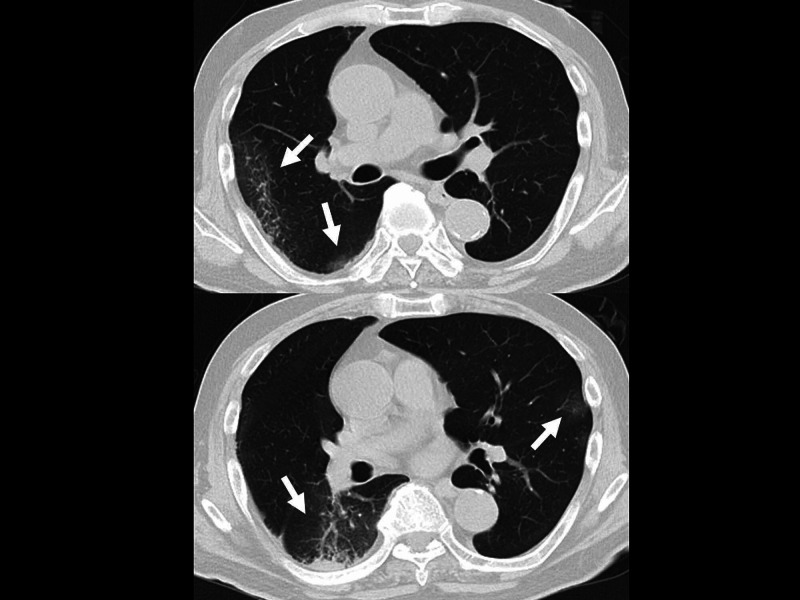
Thoracic computed tomography CT revealed bilateral local peripheral ground-glass opacities (arrow) in the lung fields.
